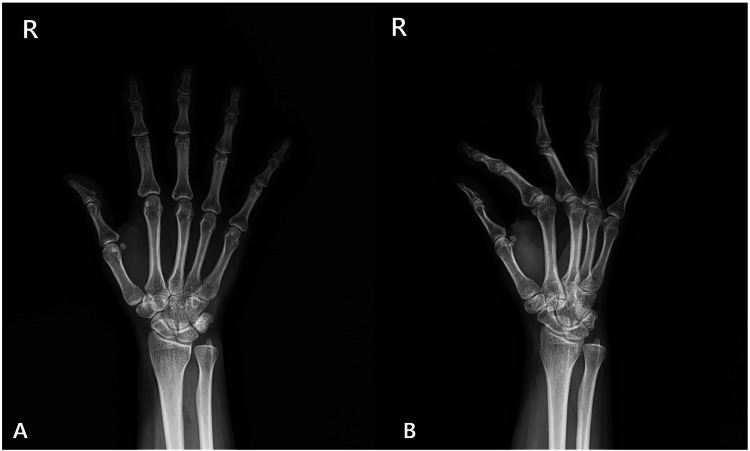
After six weeks, follow-up hand radiographs in the anteroposterior (A) and oblique (B) views demonstrated interval resorption of the calcific deposit.
